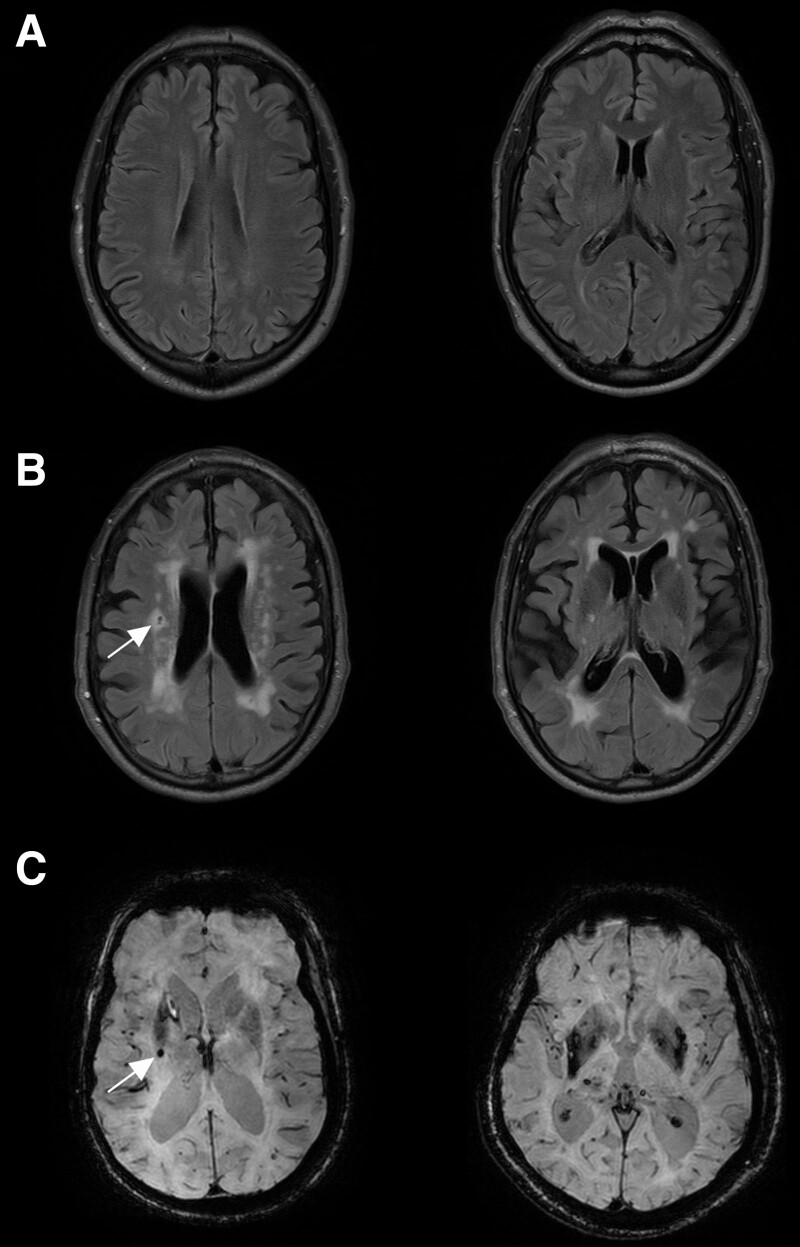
Magnetic resonance imaging (MRI) evidence of small vessel disease (SVD). A, Fluid-attenuated inversion recovery (FLAIR) MRI scans of an older patient with minimal white matter hyperintensities. B, FLAIR MRI scans from equivalent areas of another older patient with extensive white matter hyperintensities and a cavitated lacune (arrow). C, Susceptibility-weighted images from 2 different patients with SVD, showing multiple microbleeds (eg, marked with an arrow).
